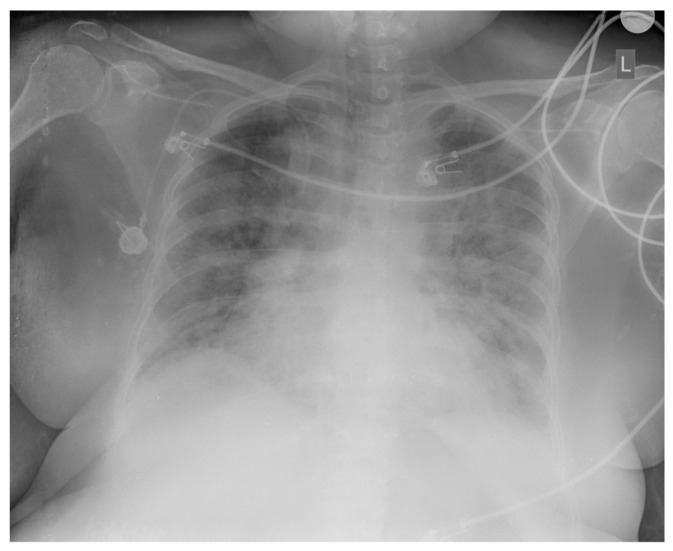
Chest X-ray: bilateral, peripheral, and lower-zone ground-glass opacities and consolidations consistent with COVID-19 pneumonia.
